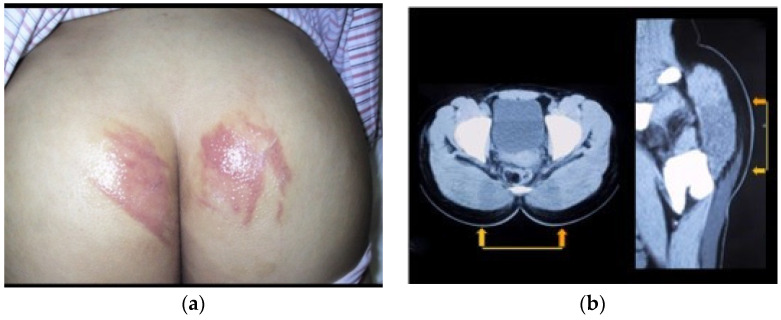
Case 2: Postoperative buttock skin lesion. (a) Clinical photograph on postoperative day 1 showing diffuse bilateral buttock swelling. A linear erythematous lesion was observed on the right buttock, and a band-like erythematous lesion on the left. Lesions were non-blanchable on glass-plate compression. Severe pain prevented supine positioning. (b) Axial computed tomography image on postoperative day 2 showing localized edema in the buttock subcutaneous tissues and bilateral gluteal muscles adjacent to the sacrum (arrows).
